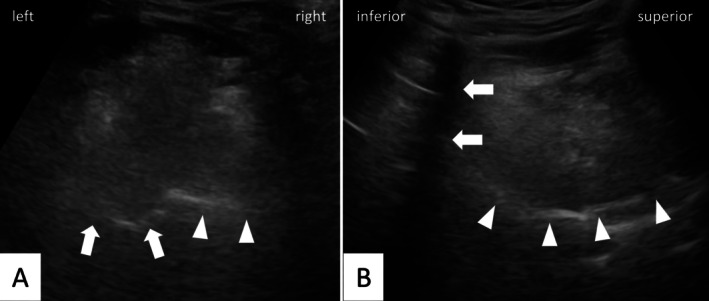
Ultrasonographic still image from the recorded sequences in the axial (A) and sagittal (B) planes of the tongue base. In the axial plane (A), the right implanted tongue base to airway interface is indicated with arrowheads, and the left non‐implanted side is indicated by arrows. On the sagittal view (B), the tongue‐airway interface is indicated with arrowheads and the hyoid shadow using arrows.
